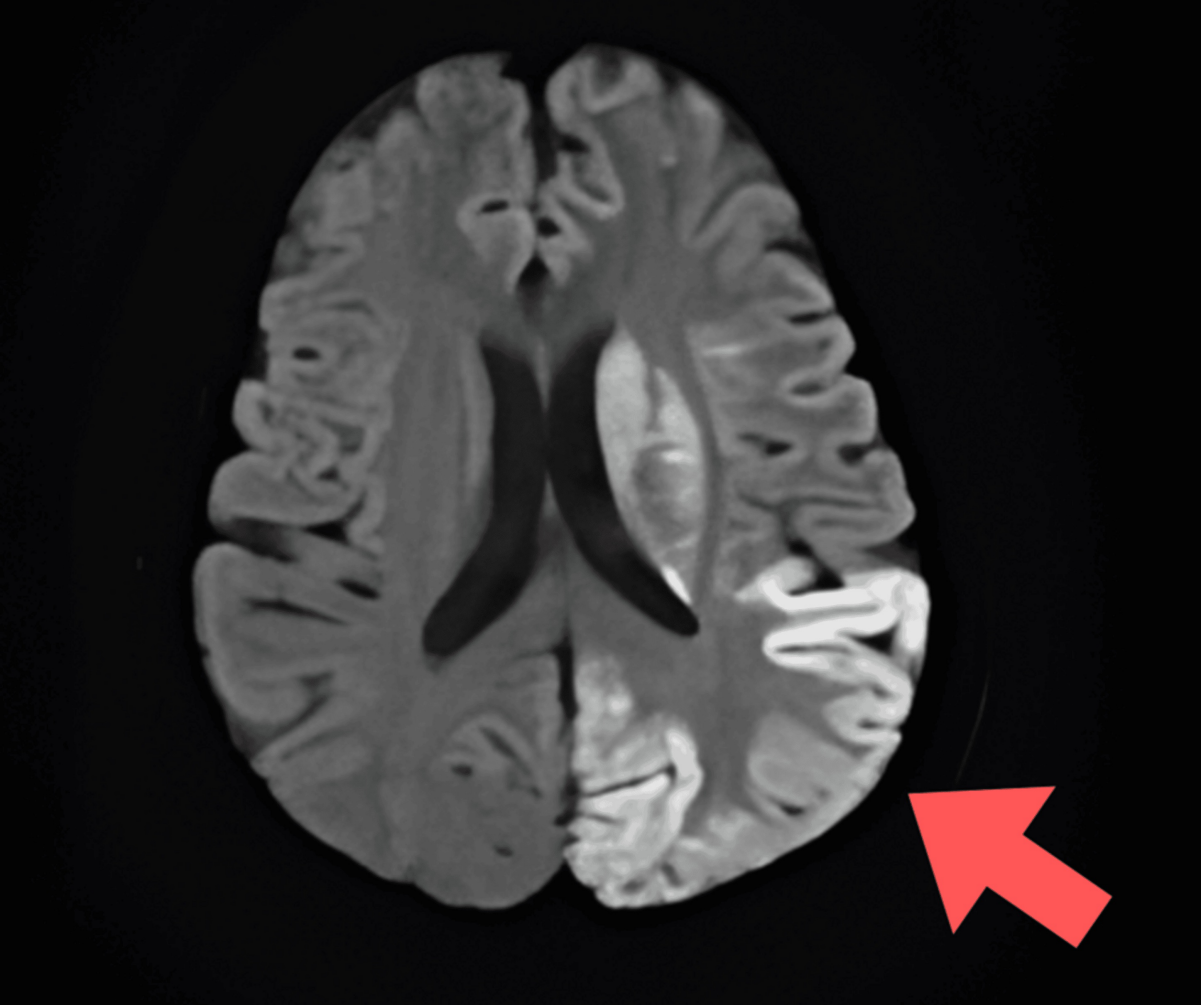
Diffusion-weighted magnetic resonance imaging (DW-MRI) showing hyperintensity in the right parieto-occipital region.
